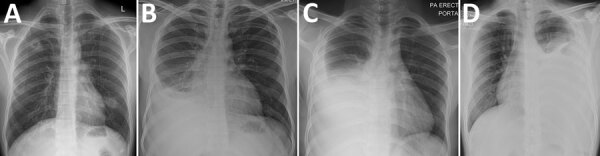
Plain chest radiographs of 4 patients with severe acute respiratory syndrome coronavirus 2 and *Mycobacterium tuberculosis* co-infection, Singapore. A) Patient 1, showing bilateral cavitary lesions; B) patient 2, showing a large right-sided loculated pleural effusion and adjacent consolidation; C) patient 3, showing a large right-sided pleural effusion with adjacent compressive atelectasis; D) patient 4, showing a large left-sided pleural effusion with adjacent consolidation.
